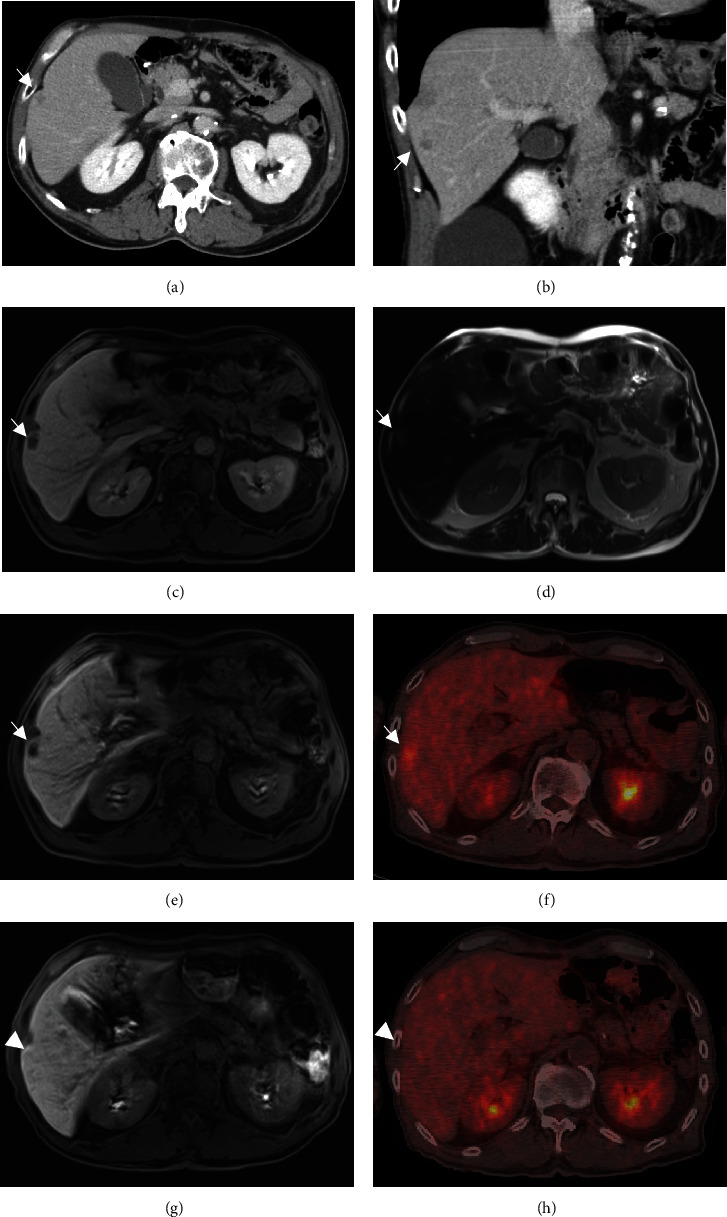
Radiological findings following liver metastasis (segment 5/6) from AFP-producing gastric carcinoma contrast-enhanced computed tomography revealing a hypovascular nodule in segment 5/6 of the liver (a, b). T1- and T2-weighted MRI showing a low-signal nodule and slightly high signal, respectively; a 9 mm defect is observed with EOB-MRI (c–e). ^18^F-FDG PET/CT showing an abnormal accumulation of ^18^F-FDG (maximum standardized uptake value of 4.8) at the same point (f). The tumor was undetectable on EOB-MRI after four courses of CapeOX therapy; no accumulation was detected on PET/CT (g, h). Abbreviations: CapeOX: capecitabine/oxaliplatin; CT: computed tomography; EOB: gadolinium ethoxybenzyl-diethylenetriaminepentaacetic acid; MRI: magnetic resonance imaging; PET: positron emission tomography; ^18^F-FDG: 2-deoxy-2-[fluorine-18] fluoro-D-glucose.
